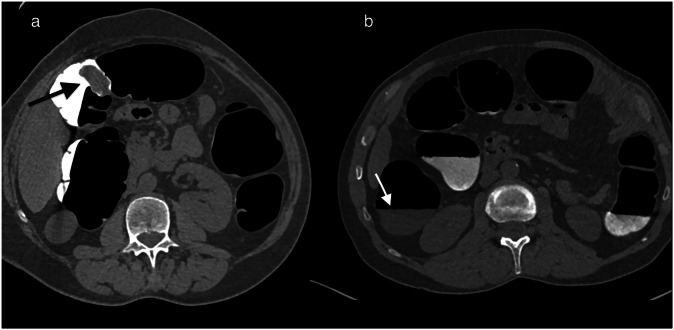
Optimal colon preparation for CT detection of colonic tumors. a Patient with state-of-the-art bowel preparation. The axial image shows the colonic lesion (indicated by the black arrow), exhibiting a tissue density that is easily detectable within an optimal radiological environment characterized by air density (< 1000 HU) and fluid tagging (> 1000 HU). b Another patient with incomplete fecal tagging. The axial slice reveals residual stool not marked by the oral contrast (white arrow), making it challenging to detect a colonic tumor
